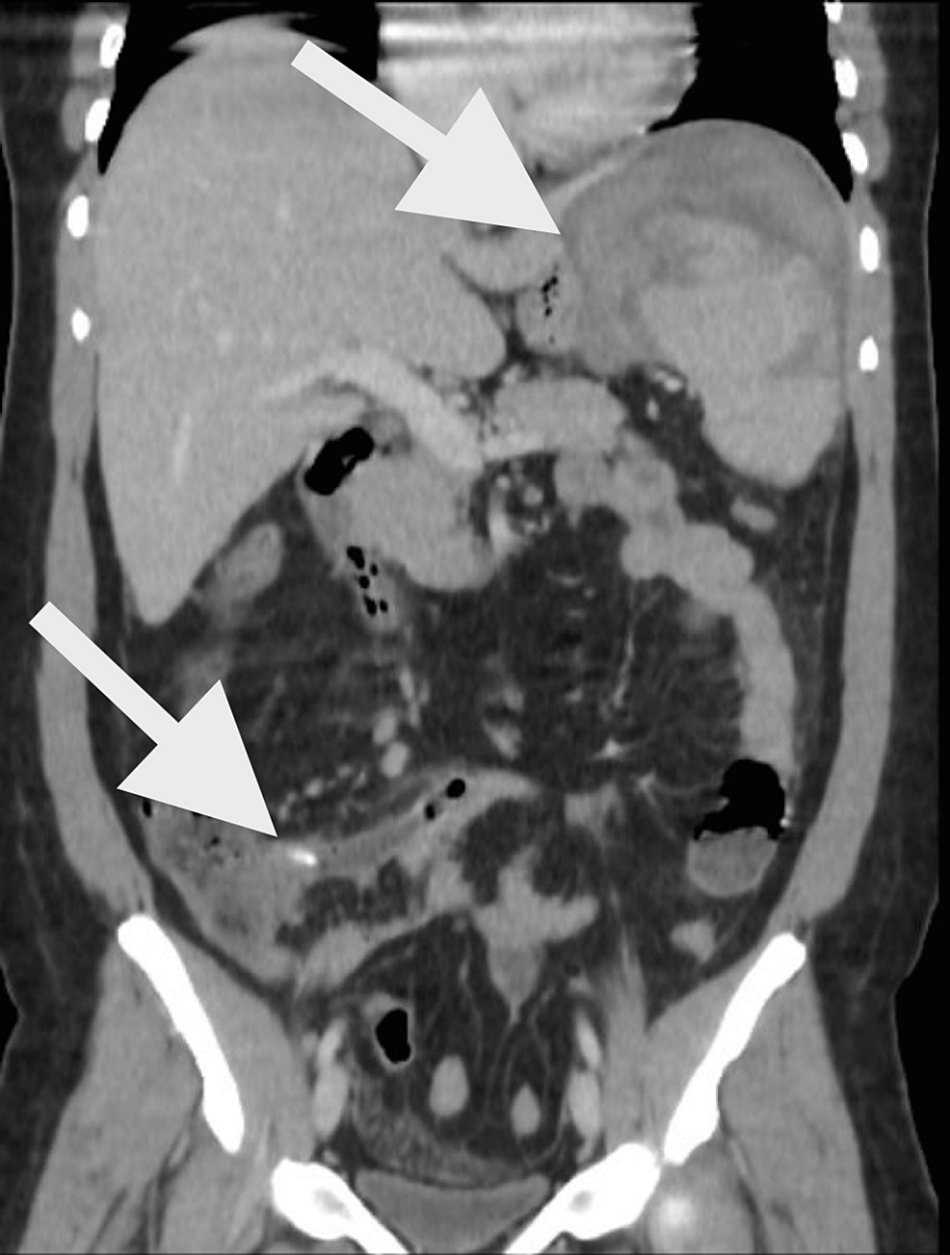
Abdominopelvic CT scan findings. The first arrow shows the grade IV spontaneously ruptured spleen. The second arrow shows the dilated appendix (15 mm) with fecalith, representing acute appendicitis.
